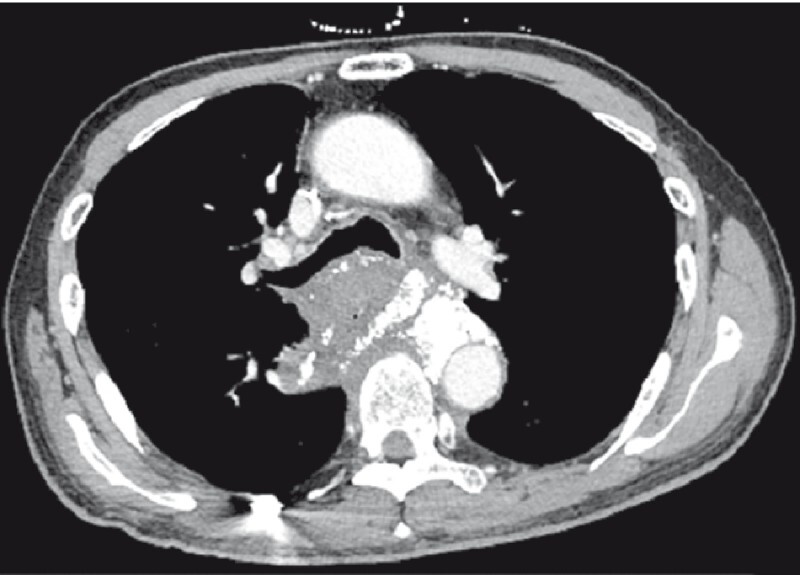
A pseudoaneurysm of the descending thoracic aorta on abdominal computed tomography angiography.
